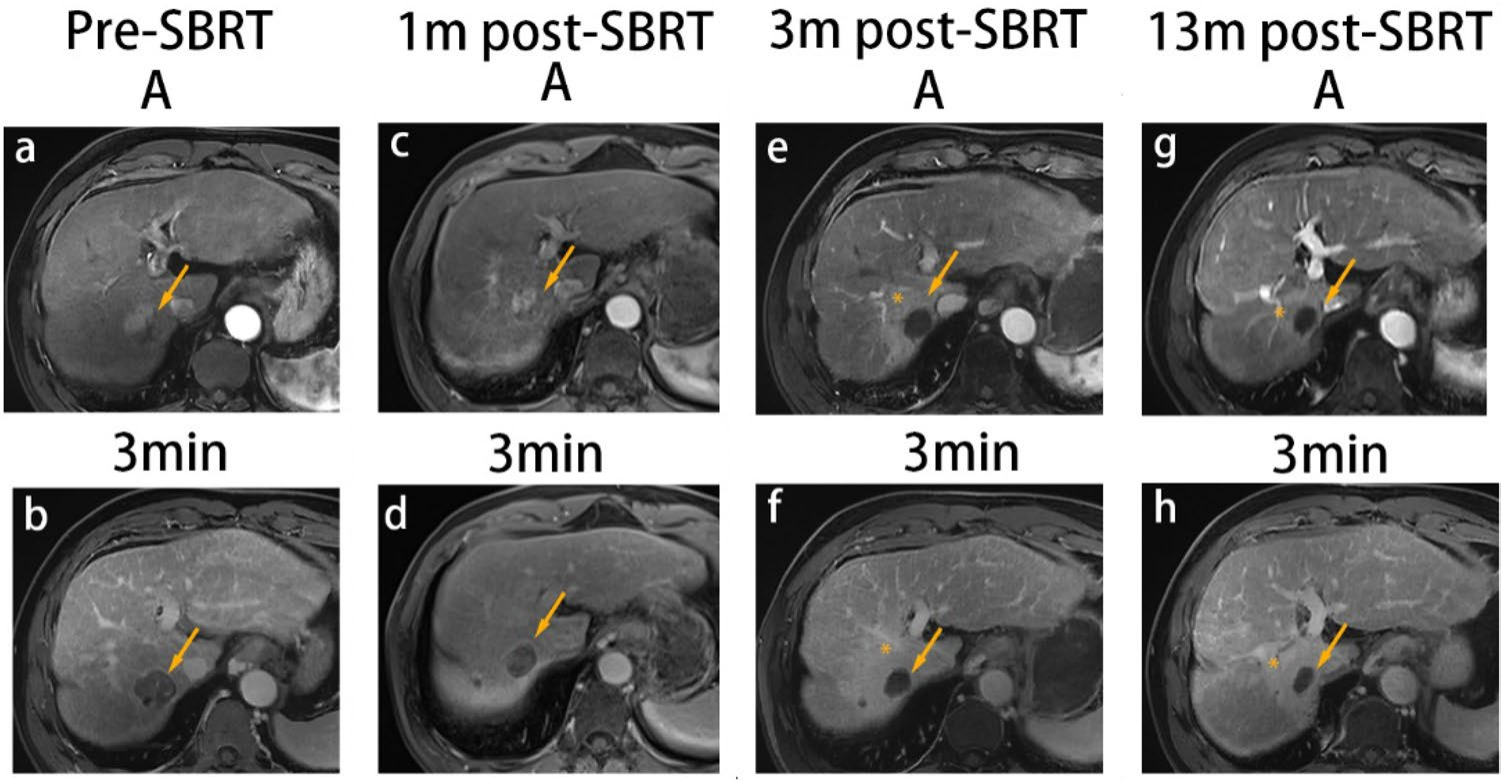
SBRT-treated HCC with conversion from APHE and washout pre-SBRT to non-enhancement immediately following SBRT. A 55-year-old man with HBV-related cirrhosis and a 3.0-cm cm HCC in the seventh segment of the liver (arrow). At baseline, the lesion exhibited APHE and washout (a, b). At 1-month post-SBRT, the lesion still exhibited APHE and washout with a decrease in size (c, d), measuring 2.5 cm. The lesion was categorized as PR and LR-TR equivocal based on m-RECIST and LI-RADS. At 3 months post-SBRT, the lesion had decreased in size, measuring 2.3 cm, with non-enhancement on multi-phased images (e, f), which persisted until the last follow-up MRI (13 months post-SBRT) (g, h). The lesion was categorized as CR and LR-TR nonviable based on m-RECIST and LI-RADS after 3 months. Additionally, at 3 months post-SBRT, there was a wedge-like parenchymal hyperenhancement with mild volume loss, which persisted until the last MRI (asterisk). *SBRT* stereotactic body radiation therapy; *A* arterial phase; 3 min, delayed phase (3 min); *PR* partial response; *CR* complete response; *APHE* arterial phase hyperenhancement; *HCC* hepatocellular carcinoma; *m-RECIST* Modified Response Evaluation Criteria in Solid Tumors; *LI-RADS* Liver Reporting and Data System; *MRI* magnetic resonance imaging
